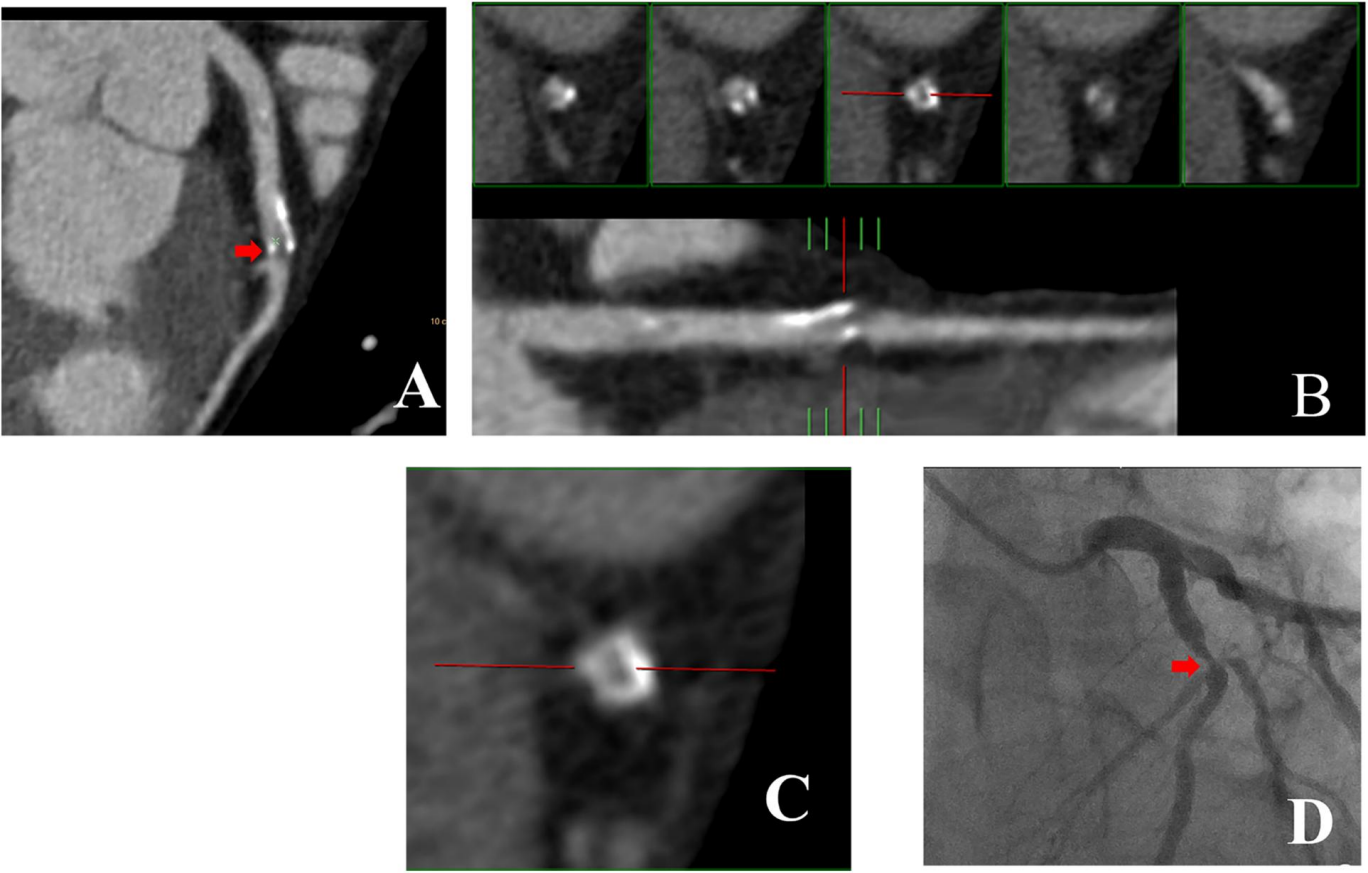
43-year-old man with R*_Hu_* = 0.38 which CCTA images shown large calcified plaque in LAD artery was corresponded to ICA. (A) Curved MPR image of LAD artery shows large calcified plaque proximally. (B) Straightened view of LAD artery lumen. (C) Enlarged view of middle small panel shown in (B). (D) ICA image of coronary artery in right anterior oblique projection shows obvious LAD artery stenosis at the position demarcated by cursor in (B,C). CCTA, coronary computed tomography angiography; ICA, invasive coronary angiography; R_*Hu*_, ratio of HU values between calcified plaque and blood; MPR, curved multiplanar reformation; LAD, Left anterior descending.
